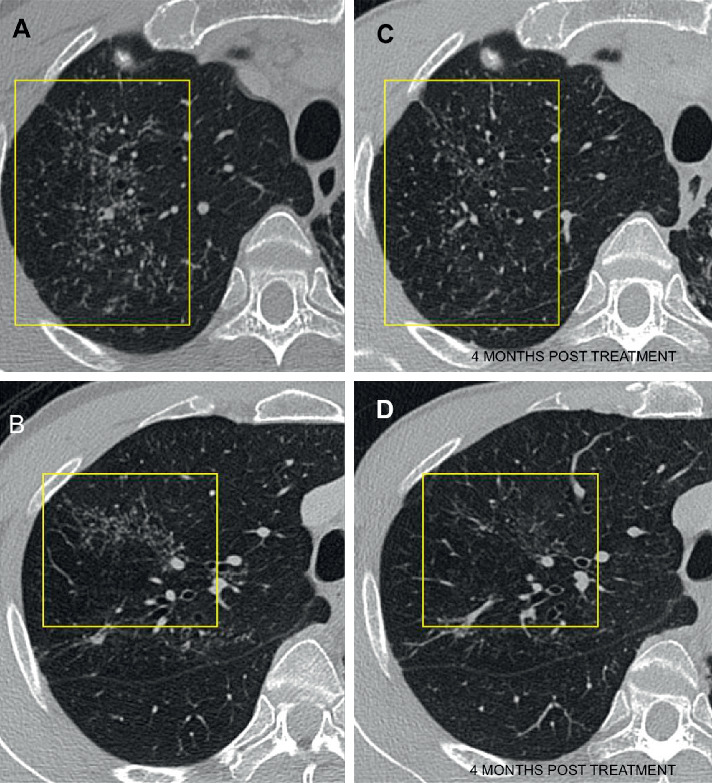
Selected computed tomography (CT) images on axial plane demonstrating clusters of micronodules with no confluence in the right upper lobe (a, b). A repeat CT (c, d) at 4 months after treatment shows improvement of the changes indicating response to anti-TB treatment.
